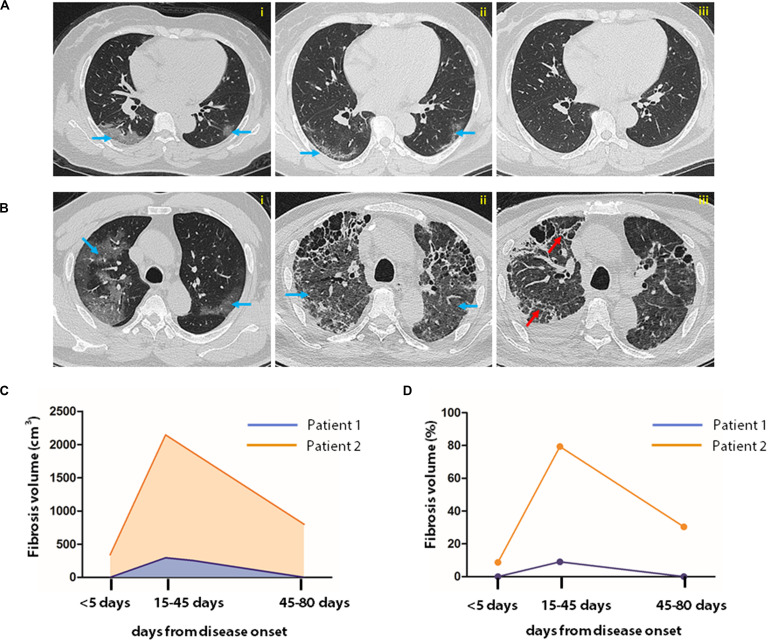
AI-assisted quantification of pneumonia lesions in COVID-19 patients. Sequential CT images of a patient with non-severe (A) and that of another with severe case of COVID-19 (B). In the patient with severe COVID-19, CT images were obtained on the 4th, 14th, and 43rd days after disease onset (A,i–iii). In patients with critical illness, CT examination was performed on the 1st, 43rd, and 80th days after disease onset (B,i–iii). Fibrosis index was computed by AI system to represent fibrosis. Fibrosis volume and percentage in the entire lung are shown in images (C,D). The blue and red arrows indicate typical SARS-CoV-2 infection-induced ground glass opacity and fibrosis images, respectively.
